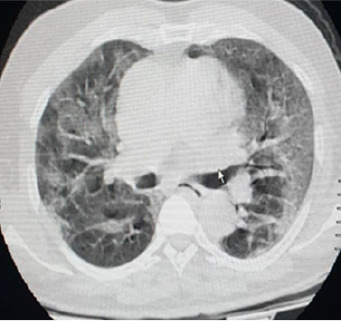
Thoracic CT showed peripheral involvement with diffuse frosted glass opacities
